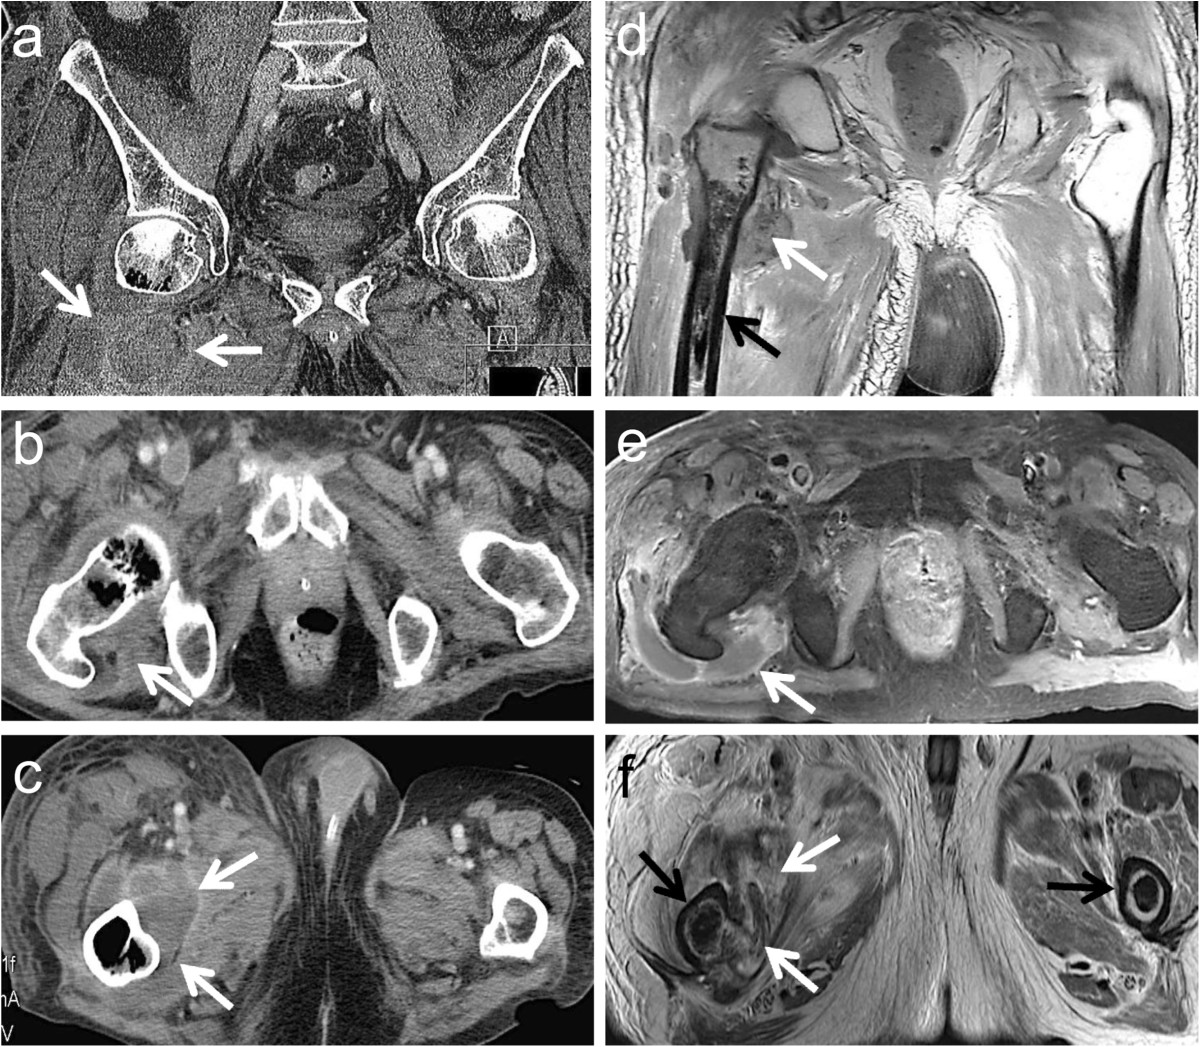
Radiological assessment of the right hip showing large intramedullary cystic lesions with soft tissue abscesses. Multipanel with coronal (a, d), proximal transverse (femoral neck; b, e) and distal transverse (lesser trochanter; c, f) CT (a, b, c) and T2-weighted MRI (d, e, f) views focusing the right hip region. Large cavitary lesions with invasion of soft tissue corresponding to abscess formation (white arrows). Underlying bone marrow osteonecrosis of both femurs (black arrows).
